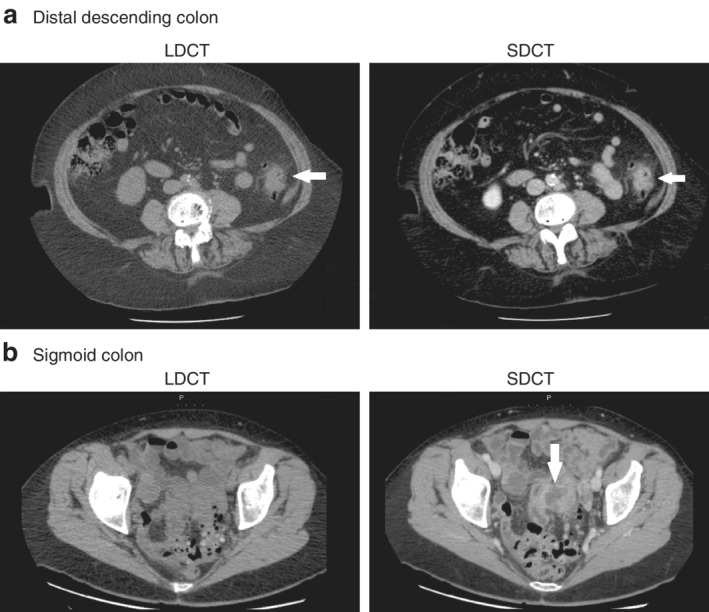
Diverticulitis diagnosed using the two CT protocols
